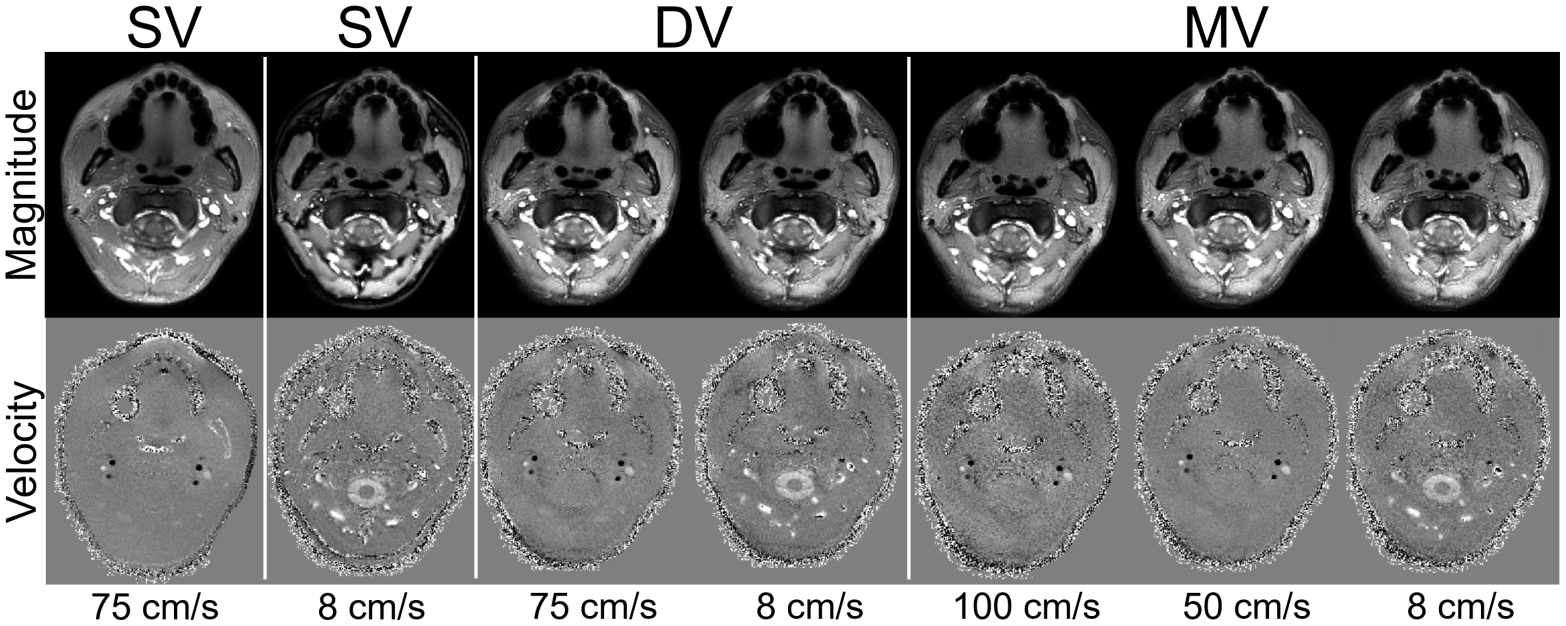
Example of magnitude (top) and velocity (bottom) images reconstructed from four 2D PC MRI scans using different flow encoding schemes in a study participant. (left to right) Single-venc (SV) scans (75 and 8 cm/s) acquired separately, and the proposed interleaved dual-venc (DV) (75 and 8 cm/s) and multi-venc (MV) (100, 50, and 8 cm/s) scans. Good subjective image quality and conspicuous depiction of the internal carotid arteries, vertebral arteries, internal jugular veins, and spinal canal were observed across scans including the DV and MV scans despite their longer echo times (TE) (SV TEs = 2.1 and 3.4 ms for vencs of 75 and 8 cm/s; DV and MV both with TE = 4.2 ms) and undersampling factors (1.13, 0.70, and 0.50 for SV, DV, and MV). All scans had a similar acquisition time ~1 min.
